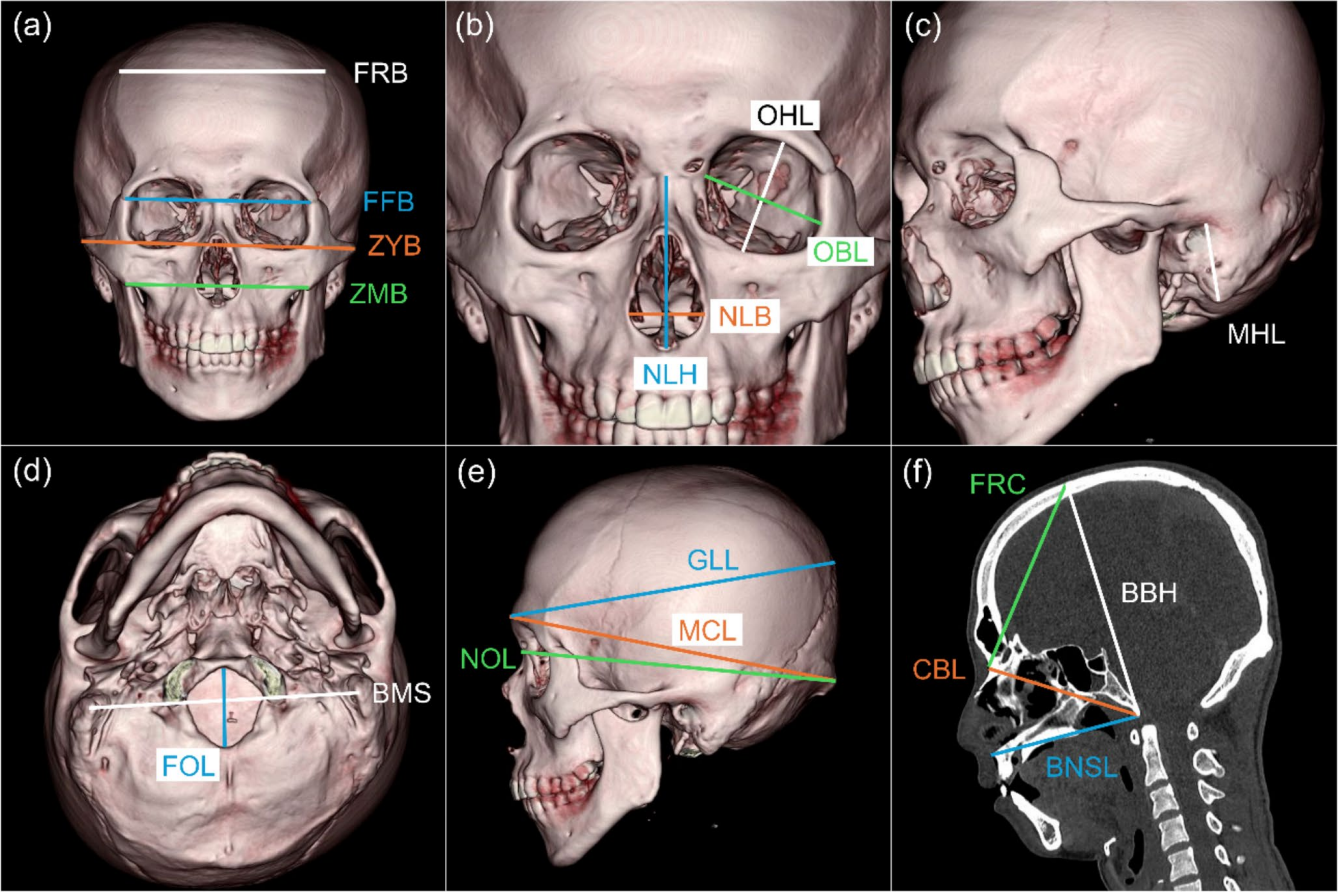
Reconstructed three-dimensional computed tomography (3D CT) images (a–e) and a sagittal plane image (f) of the skull showing measurements (see Online Resource 2 for definition); (a) from the anterior side; (b) expanded anterior side; (c) from the anterior left side; (d) from the inferior side; (e) from the left side. OHL, OBL (b), and MHL (c) were measured for each side. CBL, BBH, BNSL, and FRC (f) were actually measured using 3D CT images
